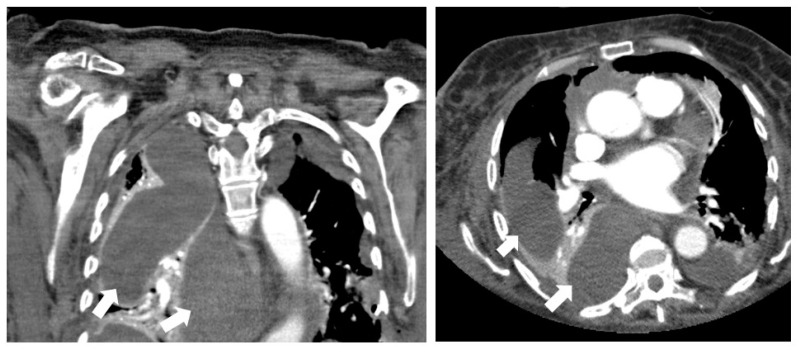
An 83-year-old woman with chambered pleural effusion (→) and CT attenuation of 8 HU. After percutaneous drainage, no pathogen was detected by microbiological approach.
